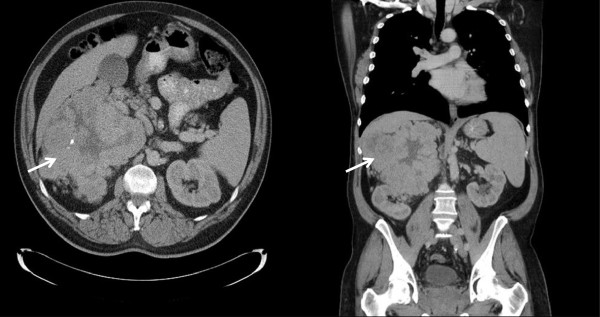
Non contrast Computed Tomography (CT) of chest, abdomen and pelvis; transverse and coronal views.
